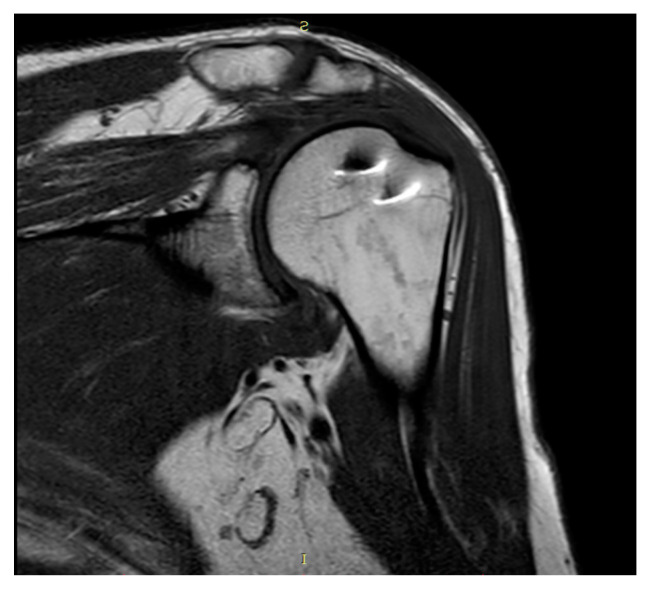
Coronal T1-weighted MRI.
